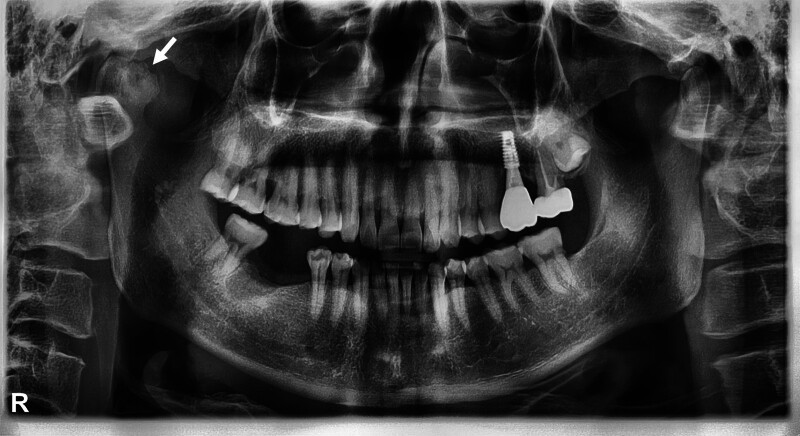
Panoramic radiograph at initial visit. The patient presented with complaints of tooth loss in the right mandibular molar region (#46, #47). An inferiorly positioned right condyle with a pedunculated, irregular mass (arrow) is visible.
